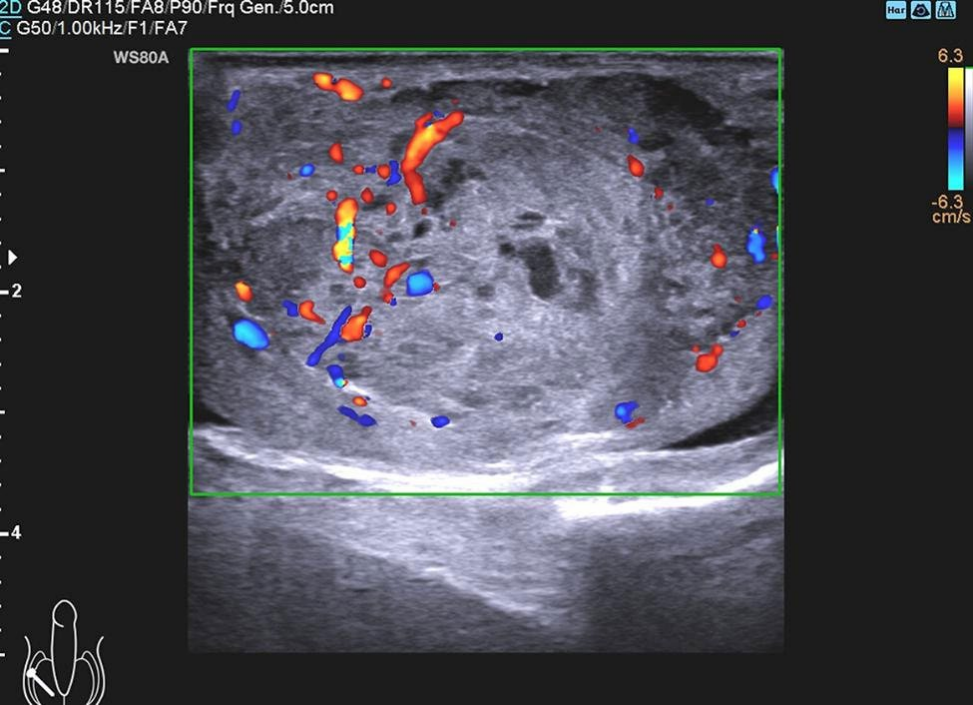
Ultrasonography showing a well-defined hypoechoic, heterogeneous mass in right testicle with internal rich flow on color Doppler.
